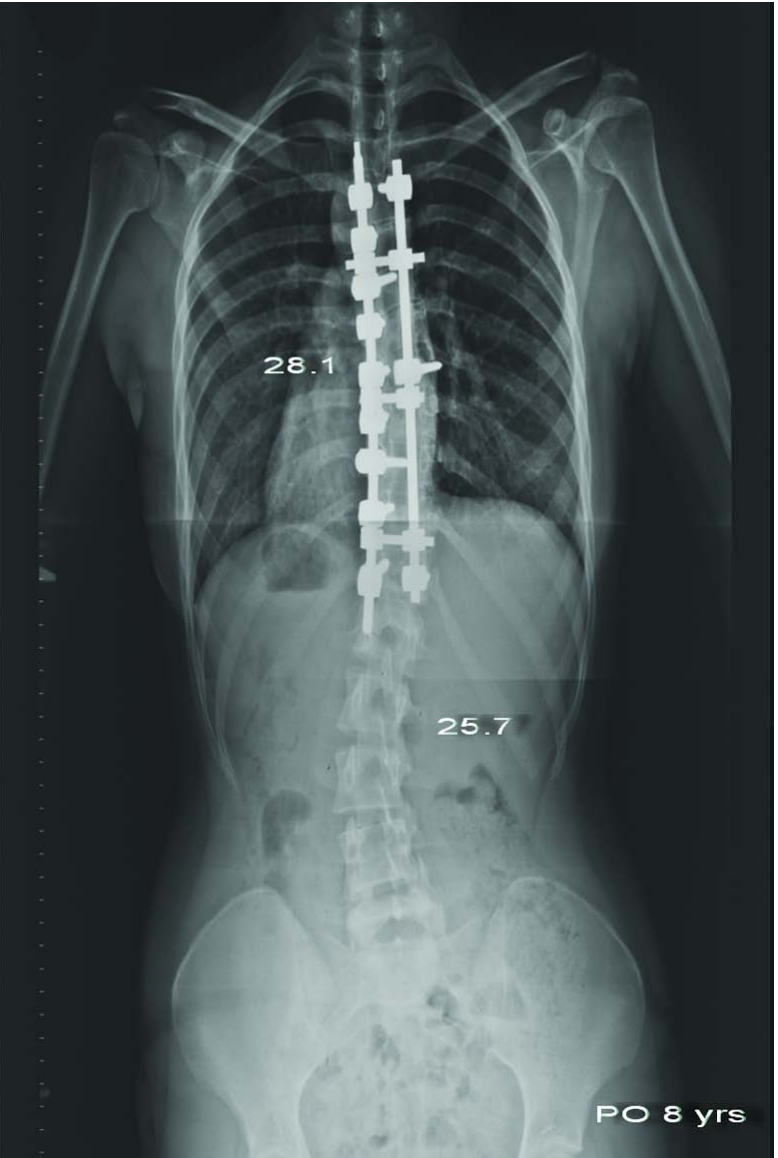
Anteroposterior radiograph of the same patient taken 8 years after surgery. The main thoracic curve was 28.1° and the lumbar curve was 25.7°. Coronal alignment was well maintained.
